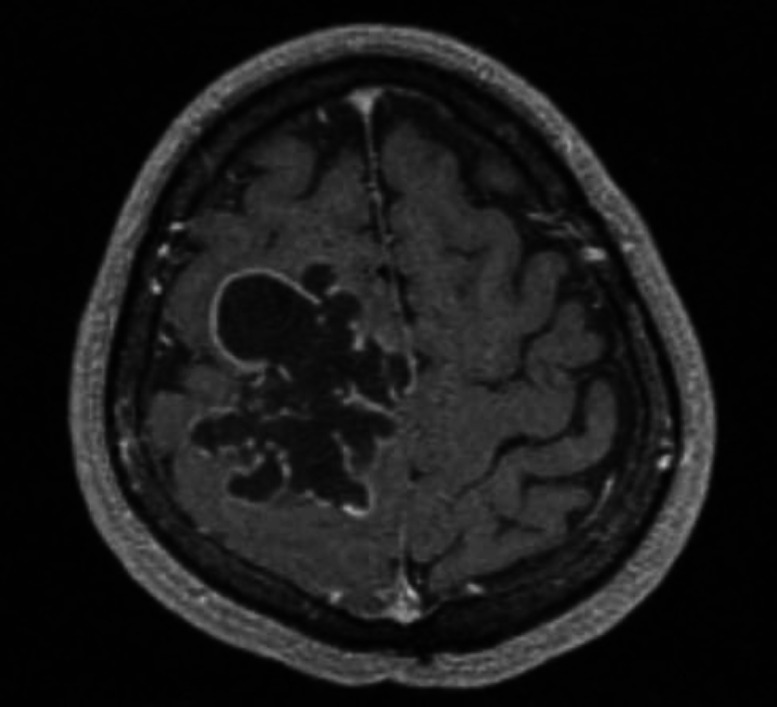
Preoperative non-contrast T1-weighted images revealed a lobulated hypointense mass with peripheral rim-like enhancement
